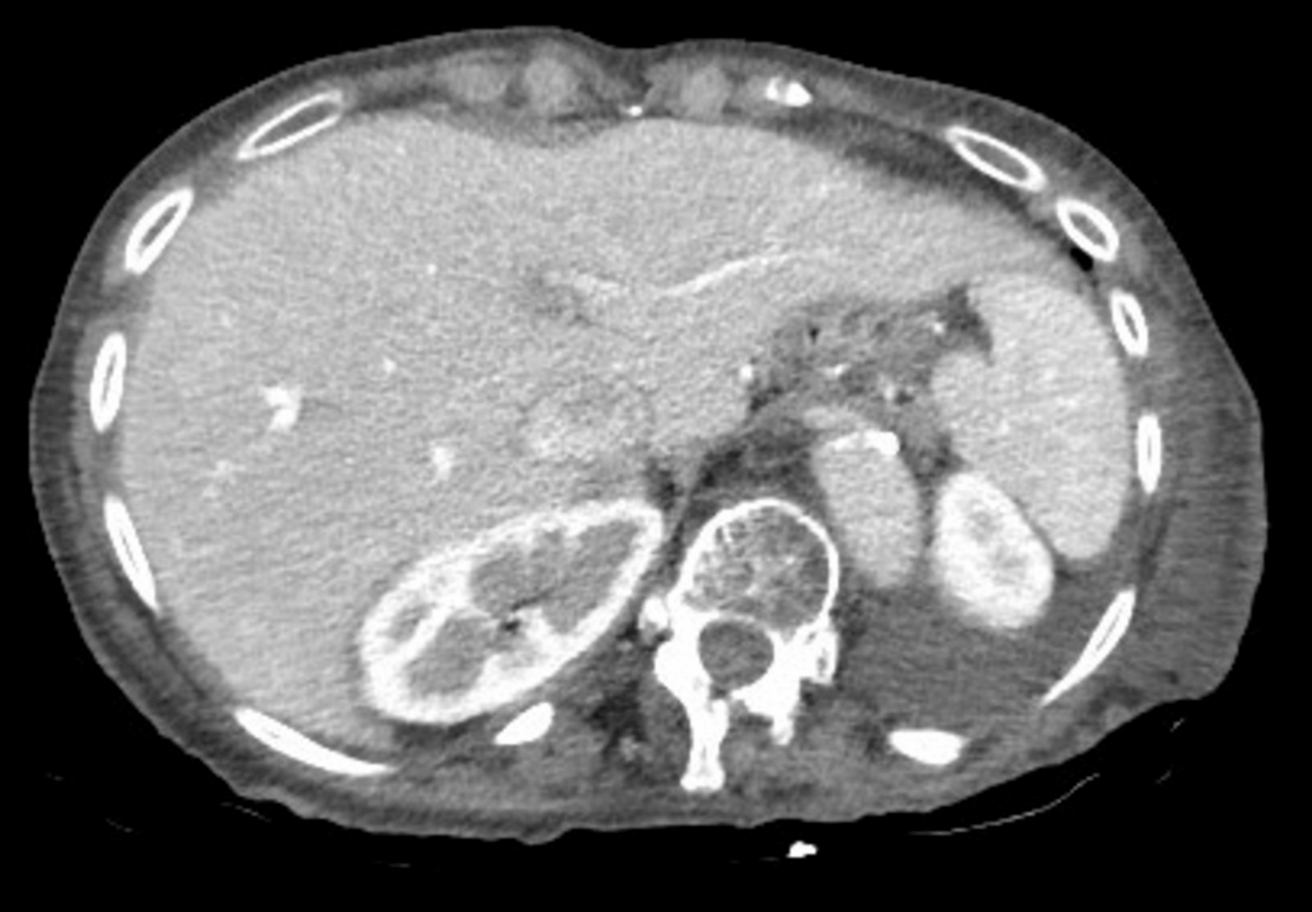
Contrast-enhanced CT of the abdomen and pelvis revealed no intrahepatic or common bile duct dilatation and a normal liver appearance.
